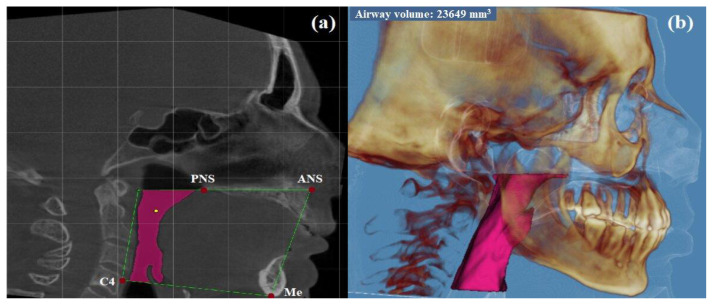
Evaluation of pharyngeal airway volume in sagittal CBCT images (a) Determination of pharyngeal airway boundaries in CBCT’s sagittal section. (b) 3D imaging of the pharyngeal airway volume CBCT, cone beam computed tomography; ANS: anterior nasal spina; PNS: posterior nasal spina; C4: supero-anterior edge of fourth cervical vertebra; Me: menton.
